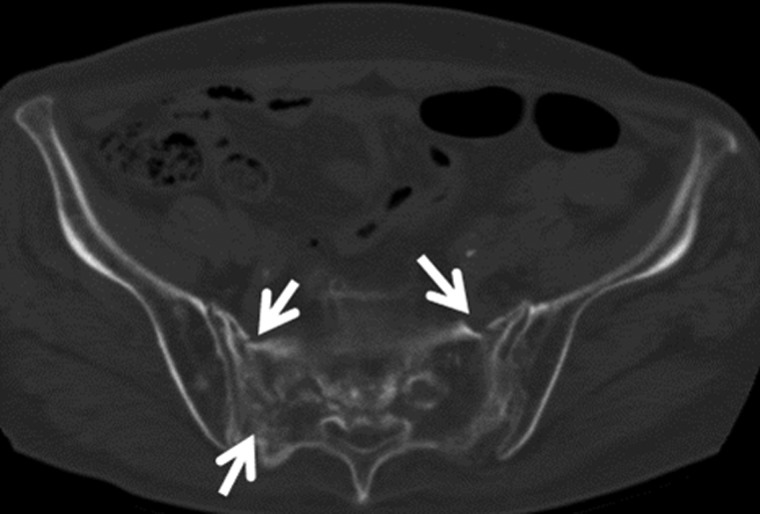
A follow-up CT image obtained 12 months after treatment shows multiple sacral fracture lines (arrows).
